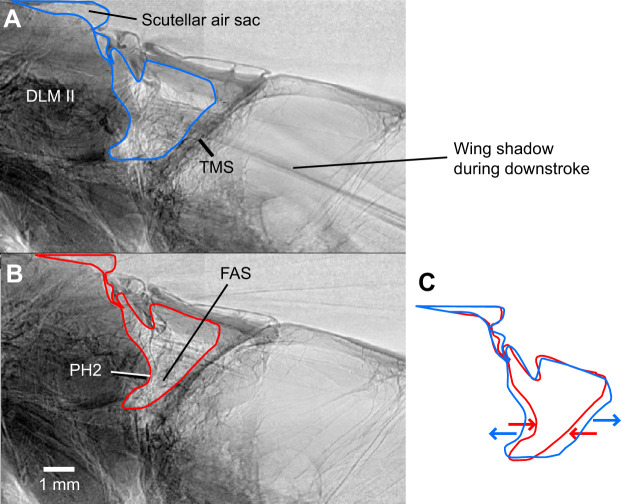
Radiographs of the mesophragma shift inducing volume changes in the fused metathoracic–abdominal air sacs (FAS) by contraction and relaxation of the longitudinal flight muscles. (A,B) Radiographs from the sequence of a tethered *Acherontia atropos* (male, 1/05) (Movie 1). Lateral view. (A) During downstroke of the wings, the contracting dorsolongitudinal muscles (DLM II) pull the mesophragma (PH2) in the anterior direction, widening the FAS under coordinated abdomen lifting (blue contours). (B) During upstroke of the wings, under contraction of the more lateral dorsoventral muscles, the relaxing DLM II allow backward shift of the PH2, reducing the space of the FAS (red contours). (C) Changes of bounds of the FAS, red and blue arrows indicating direction of volume change (redrawn from A and B). TMS, transverse muscle septum.
